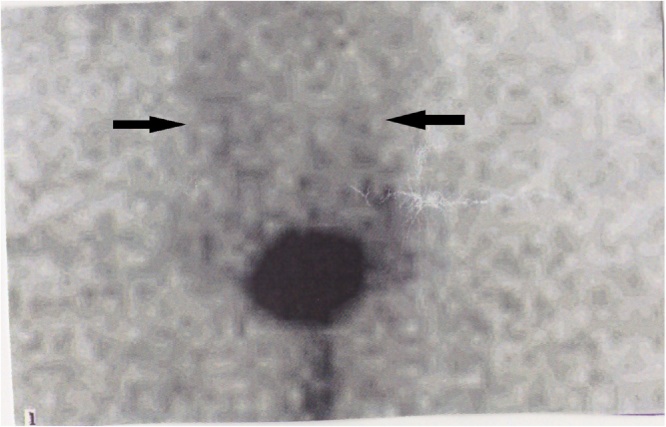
Delayed lymphoscintigraphy image showing no uptake in bilateral inguinal lymph nodes (arrow).
